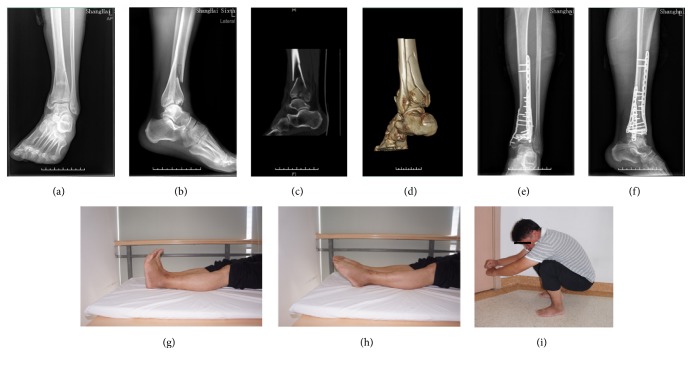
The image and functional outcome of a typical patient. Preoperative imaging: (a, b) X-ray; (c, d) CT. Postoperative imaging: (e, f) X-ray. The range of ankle movement: (g) dorsiflexion; (h) plantarflexion; (i) squat.
